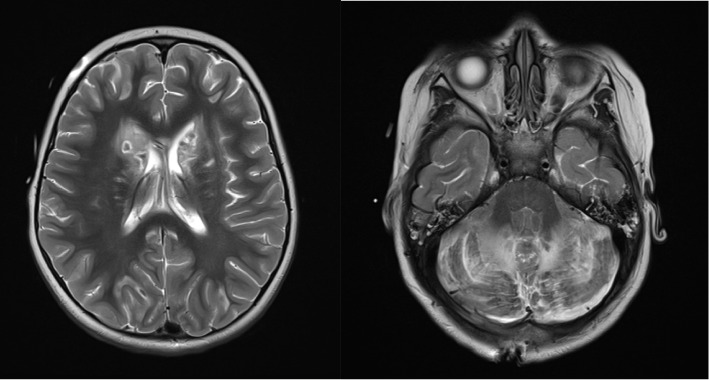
Prognostic MRI 16 days postingestion showing evolution of extensive multifocal signal abnormality. There is progressive necrosis/cavitation of the caudate heads, anterior putamen, hippocampi, and cerebellar hemispheres. Persistent diffusion restriction within the globi pallidi and new involvement of the subthalamic nuclei bilaterally.
